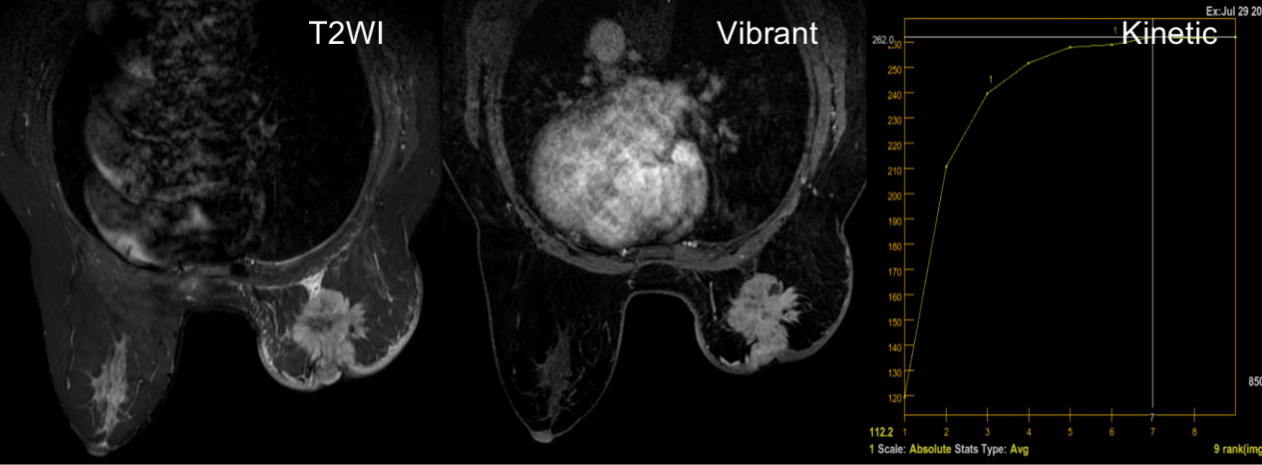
A 53-year-old woman diagnosed with invasive ductal carcinoma of the luminal B subtype. The T2-weighted image shows a strong hyperintense signal inside the mass without enhancement on subtracted images, representing necrosis. The Vibrant technology shows an irregular mass with an irregular margin and a heterogeneous enhancement. Kinetic curves generated from two regions of the enhanced ring demonstrate a plateau appearance (type II curve).
